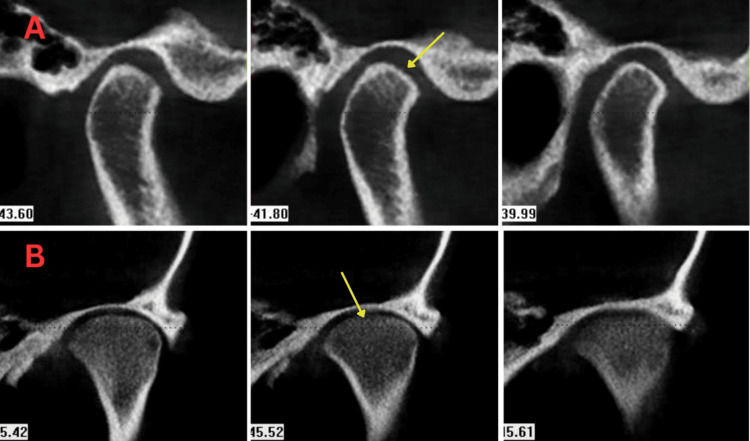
CBCT imaging showing TMJ grade 1. A: Mild flattening of contours. B: Mild thinning of cortical outline. CBCT: cone-beam computed tomography; TMJ: temporomandibular joint.
